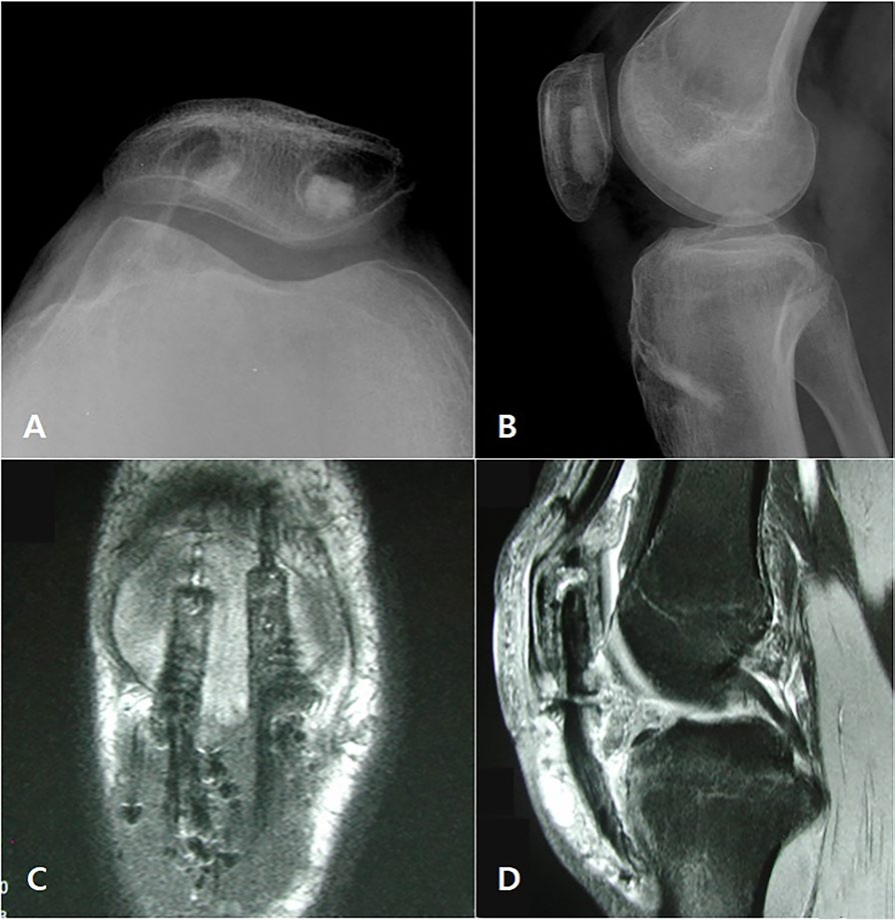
Postoperative images. a Skyline view of plain radiograph (b) Lateral view of plain radiograph. c T2 weighted sagittal MRI. d T1 weighted coronal MRI
